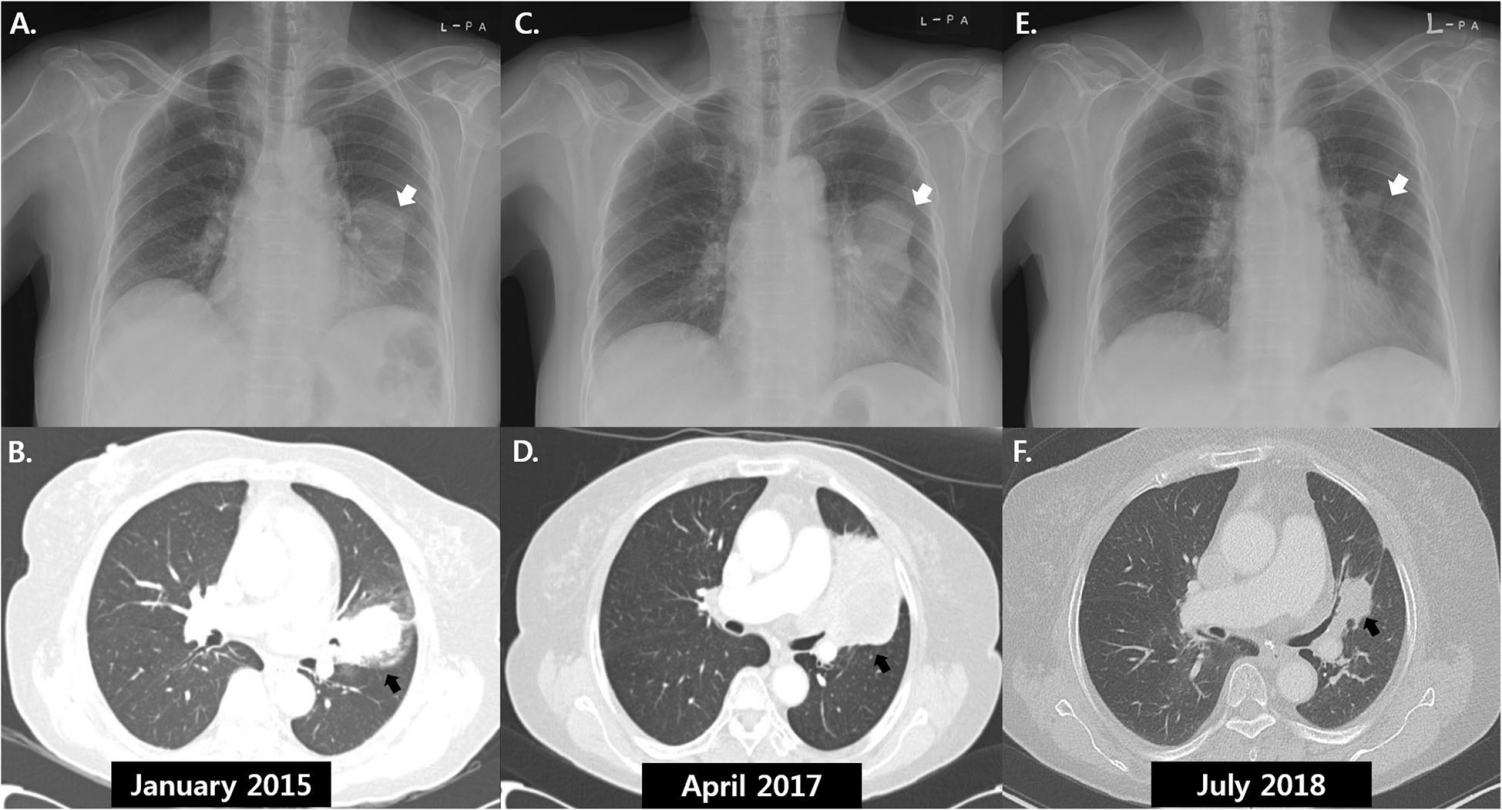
Serial chest radiographs and computed tomography scans of a 74-year-old woman with spontaneous remission of non-small cell lung cancer. a: Radiograph in January 2015 showed a left lung mass (white arrow). b: A 5.1 × 2.9 cm lung mass in the left upper lobe (black arrow) was observed on computed tomography (CT) images at the time of initial chest radiograph (a). c and d: After fifth-line chemotherapy, follow-up radiograph (c) and CT scans (d) in April 2014 showed an increase in size of the left lung mass. e and f: One year after the discontinuation of chemotherapy, significant reduction in size of the lung mass was demonstrated on follow-up radiograph (e) and CT scans (f) in July 2018
